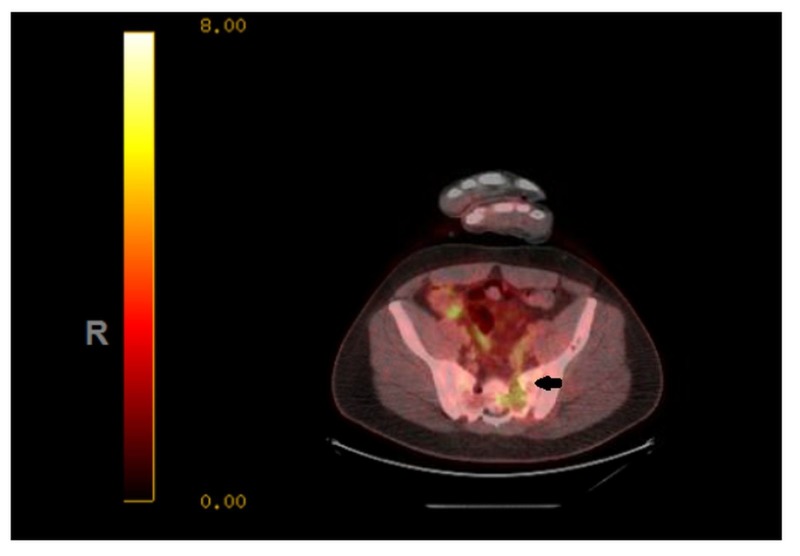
MRI scan of the pelvis (2012) - Ewing's sarcoma in left sacrum/ilium locally involving neuroforamina, paraspinous, and gluteal muscles MRI: magnetic resonance imaging
